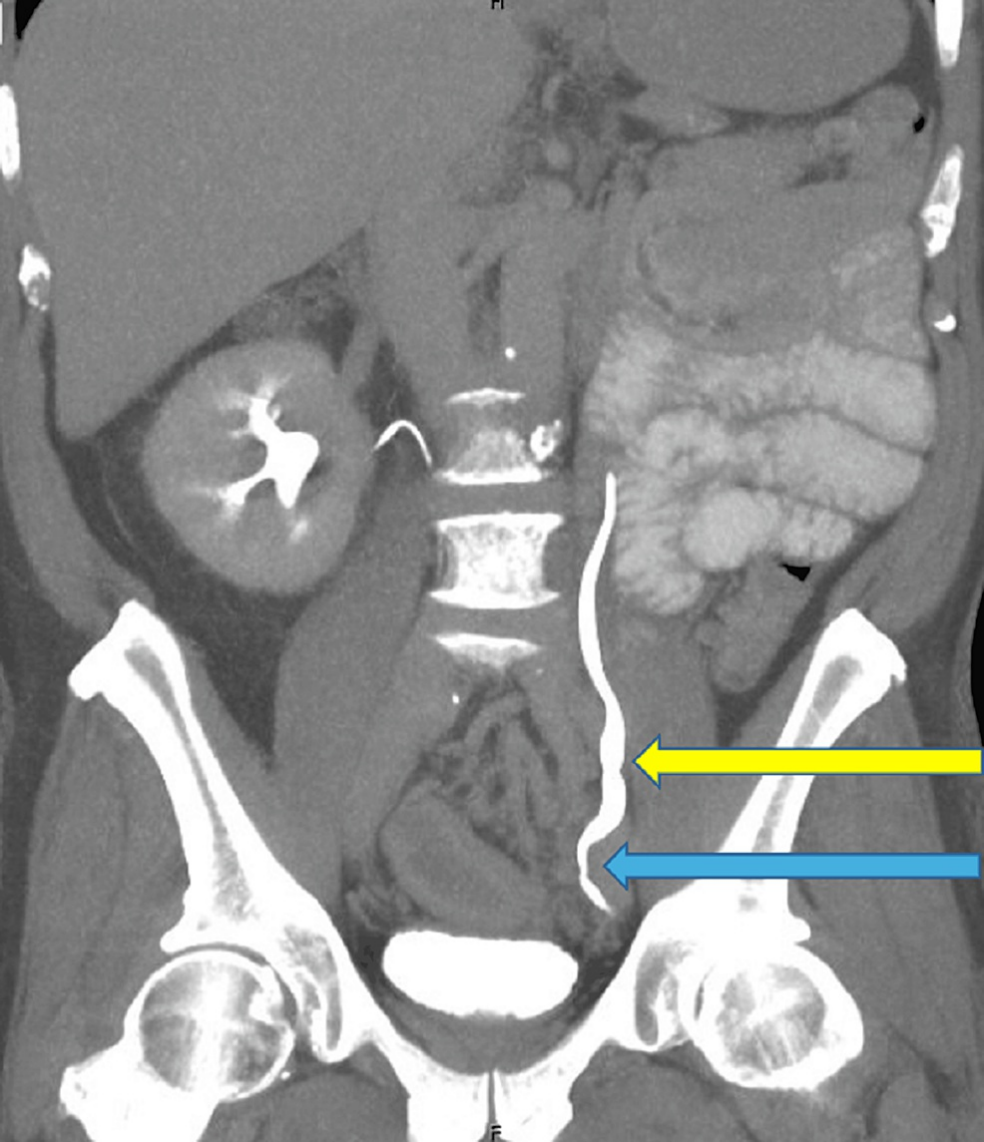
Coronal CT imaging with delayed contrast, displaying distal ureteral narrowing (blue arrow) resulting in mild hydroureter and tortuosity upstream (yellow arrow).
